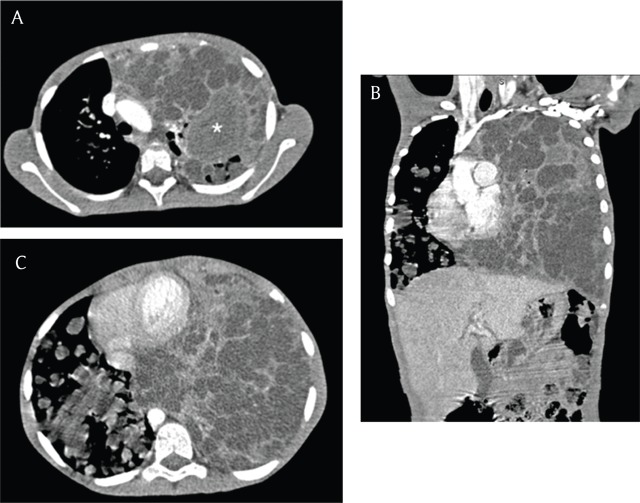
Axial and sagittal CT scan shows multiple round, cystic lesions, interspersed by atelectatic lung parenchyma on the right and fewer cystic lesions in the left lung with a residual moderate amount of aerated lung. There was one complicated cyst in the left upper lobe (Figure. 2A, asterisk). The liver shows no involvement.
